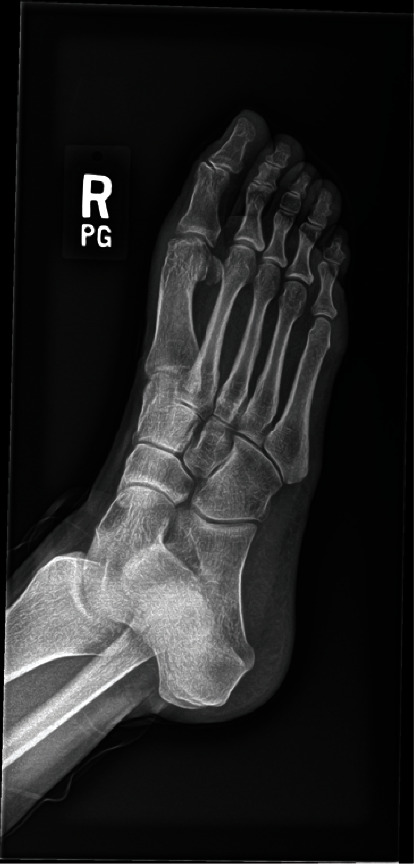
Oblique radiograph of the right foot taken three years post-OCA implantation with complete resolution of hallux rigidus.
